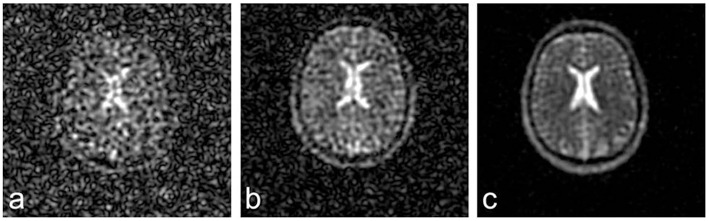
^23^NaMRI at 1.5 (A), 3 (B), and 7 T (C). Similar acquisition parameters and a nominal spatial resolution of (4 mm)^3^ were applied. SNR increases approximately linearly with magnetic field strengths. Figure reproduced from (63) with permission of John Wiley and Sons, Journal of Magnetic Resonance Imaging.
